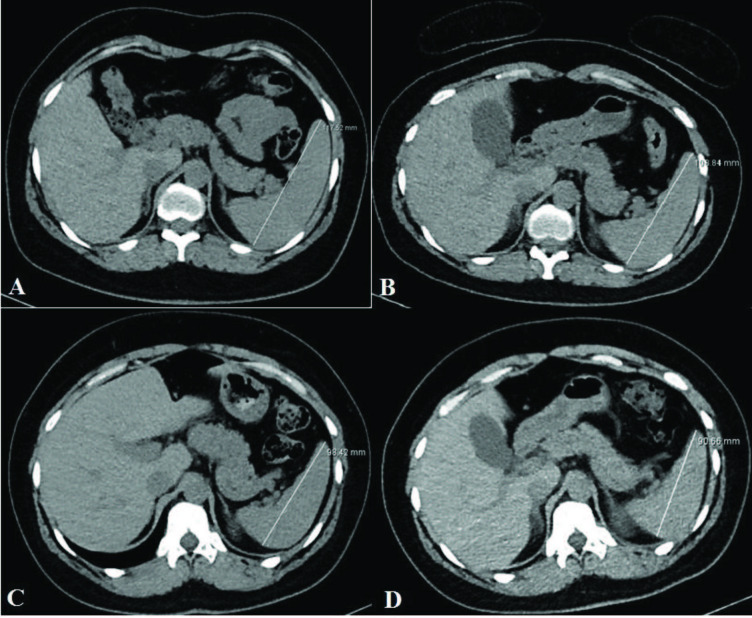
Axial nonenhanced CT showing the longest diameter of the spleen at admission [a], within 1 week [b], within 2 weeks [c], and 2 weeks after [d] the initial CT, respectively.
